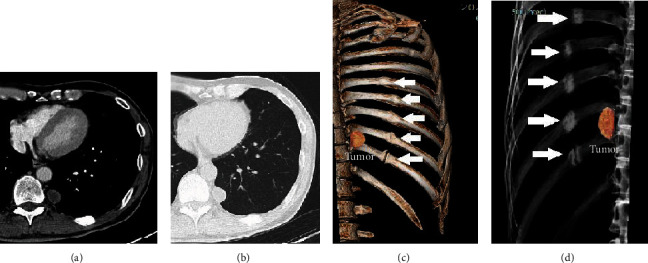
(a) Axial CT enhanced with intravenous contrast, mediastinal window. (b) Axial CT, pulmonary window. (c, d) Three-dimensional reconstruction using the CT of the chest (right ribs removed). Paraspinal oval mass in the lower thoracic spine measuring 20 mm in diameter. The capsule enhances slightly with contrast. The tumor is well-circumscribed without intraspinal extension. The callus formations in the posterior ribs (7th to 11th) can be observed, suggesting previous rib fractures (c, d; arrowheads).
